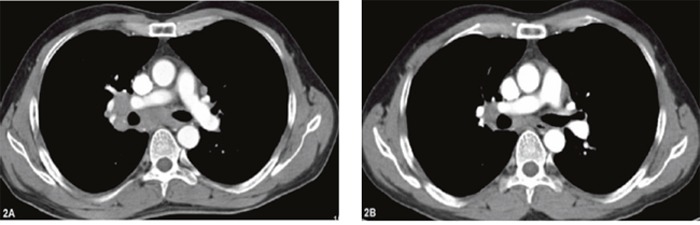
Chest computed tomography of the patient (case 1) before and after gefitinib (A) lymph node metastasis in lung hilum and mediastinum on Mar 24^th^, 2015 (before gefitinib); (B) lymph nodes shrinking on Apr 22^th^, 2015 (one month after gefitinib).
